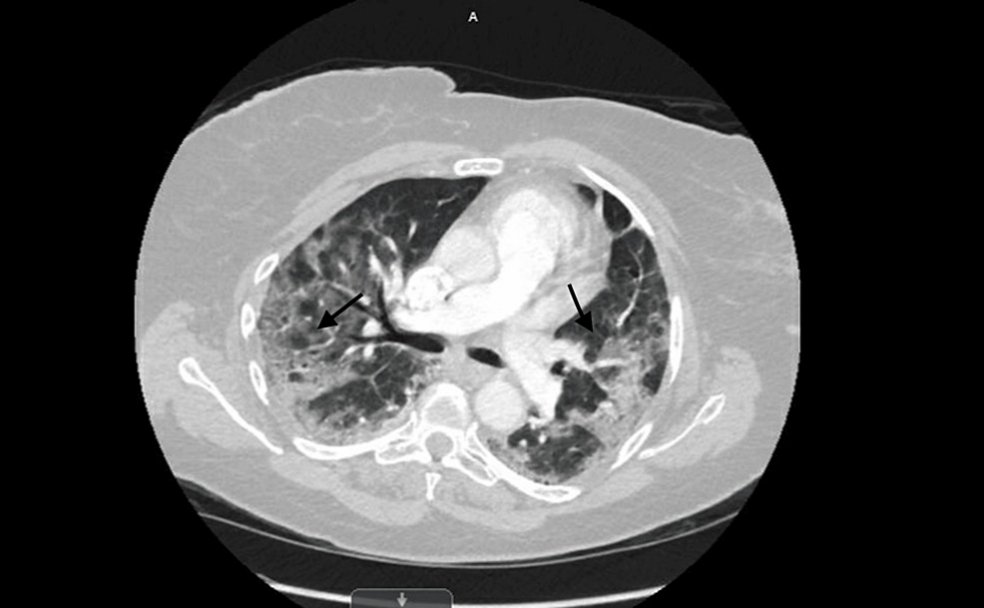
Computed tomography angiography of the chest showing bilateral patchy and ground-glass opacities (arrows).
